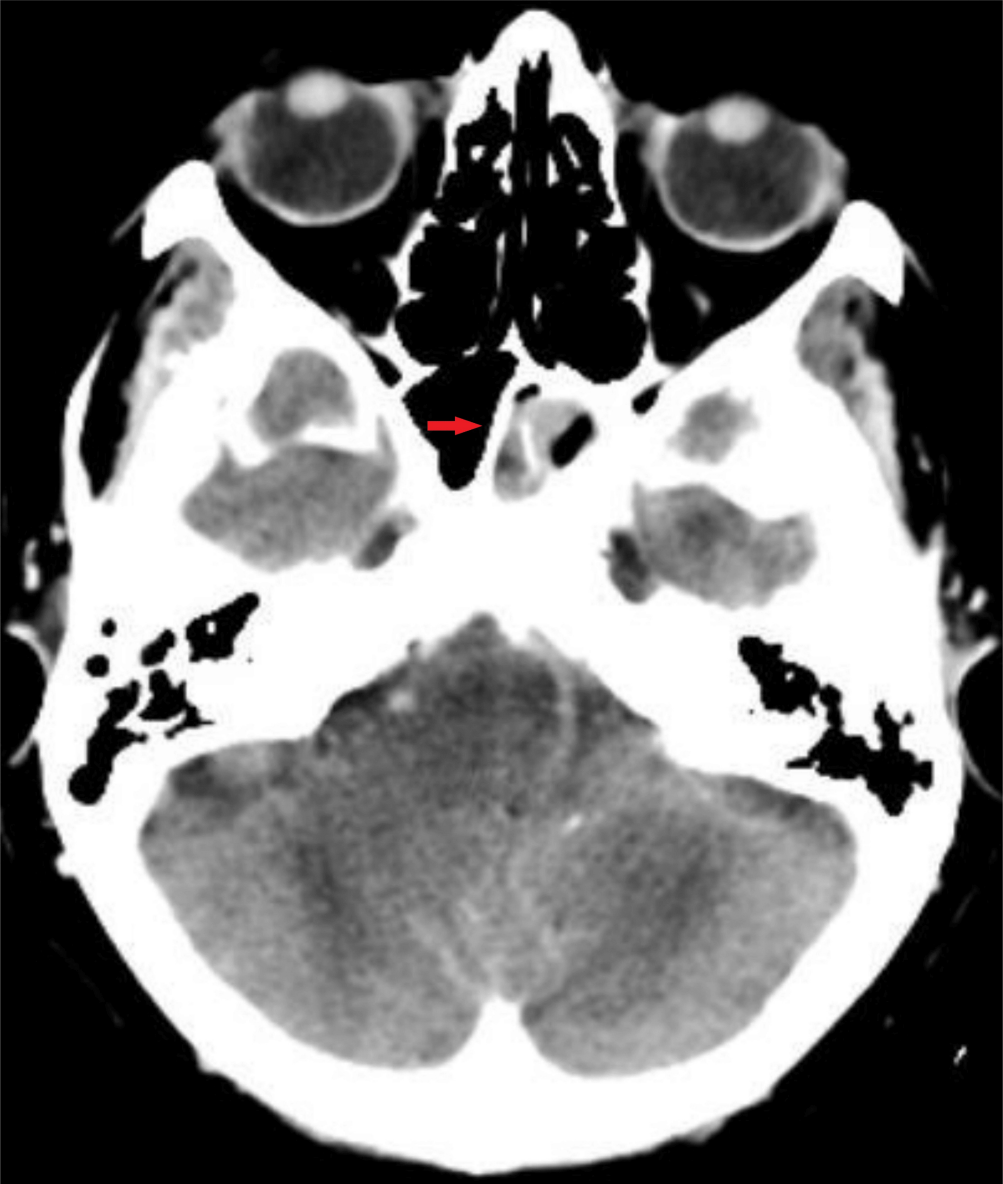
Axial view of the contrast-enhanced CT scan of paranasal sinus. The red arrow shows mucosal thickening of the left sphenoid sinus. The lesion shows enhancement from the rest of the mucosa with a calcified deposit within it. There is abnormal erosion on the floor of sella turcica CT: computed tomography
